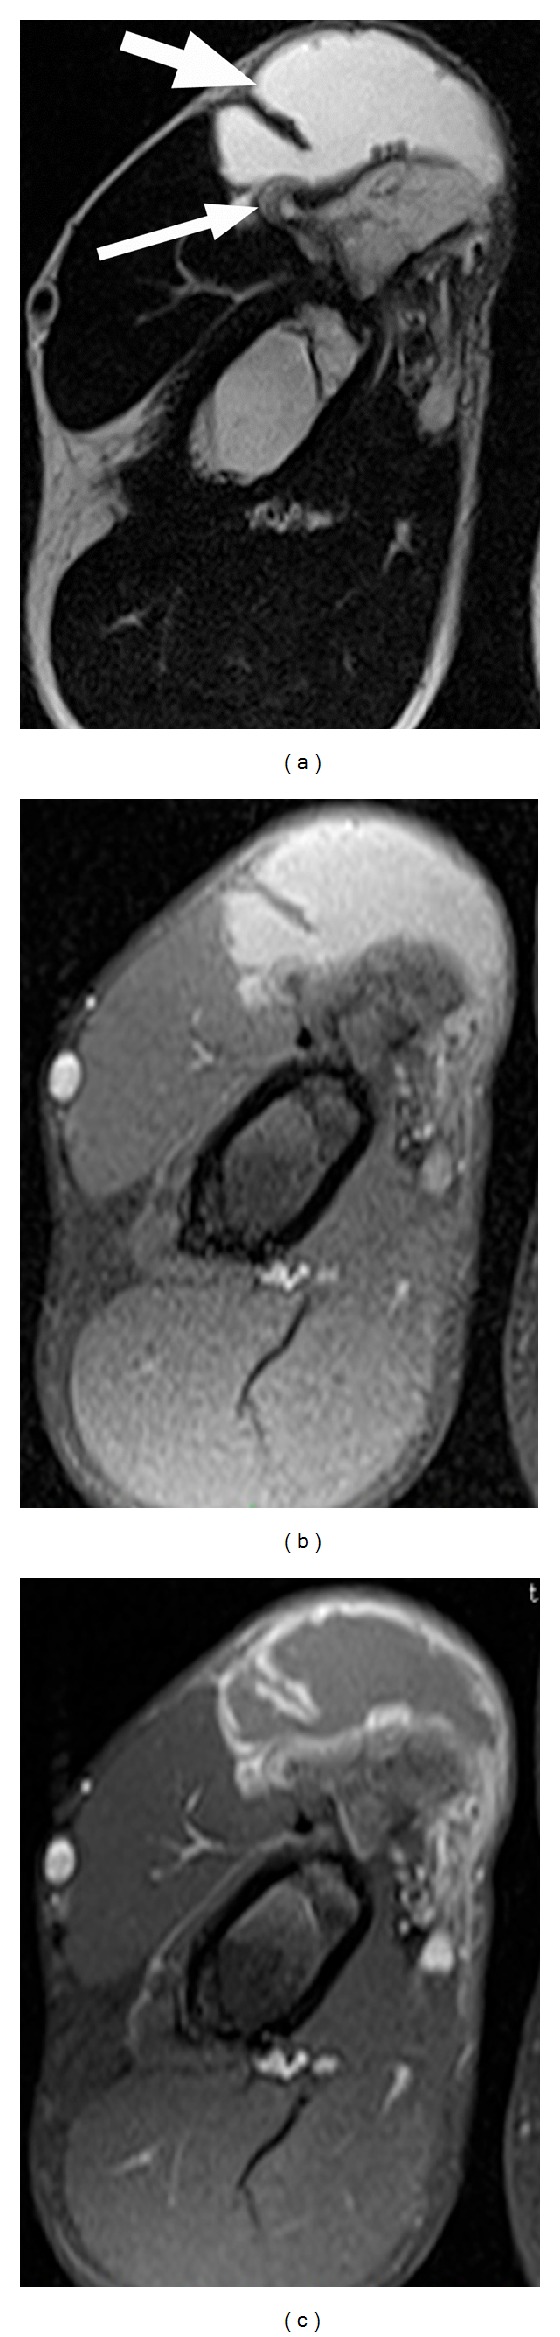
Axial T2-weighted (a), T1-weighted fat-suppressed precontrast (b), and postcontrast (c) MR images show an adventitious bursa *(thick arrow)* overlying the osteochondroma of the humerus. Note the thin cartilage cap *(thin arrow)*.
